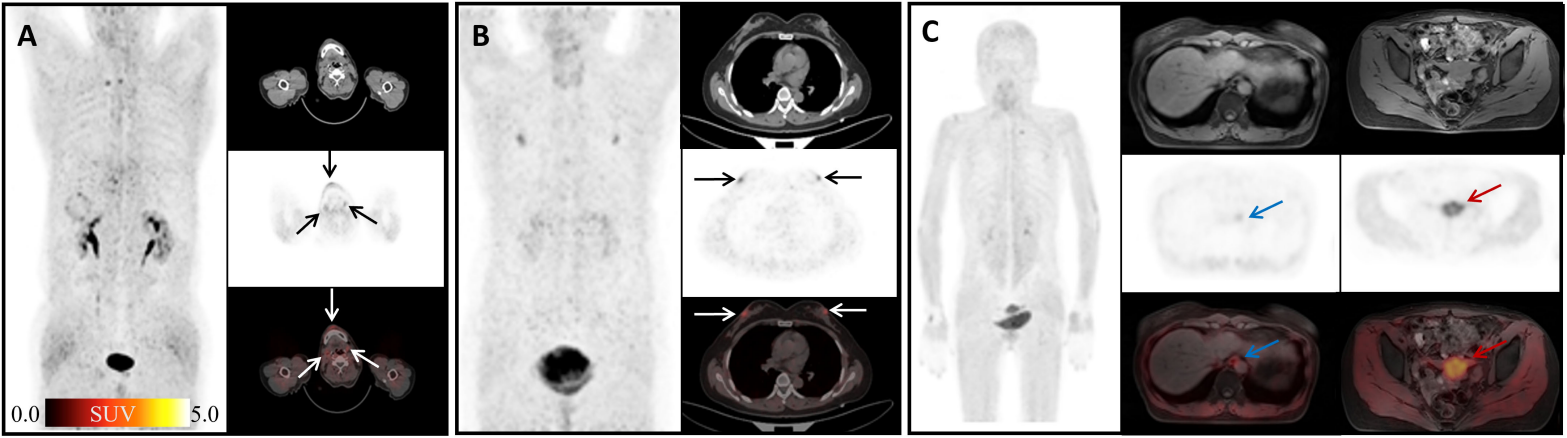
Physiological uptake of ^68^Ga-FAPI-04. (A) A 60-year-old man with hepatic hilar malignancy after the treatment of transcatheter arterial chemoembolization (TACE); the arrows indicate ^68^Ga-FAPI-04 uptake in the gingiva and submandibular gland (SUV_max_ 4.45, 3.79, and 3.64). (B) A 65-year-old woman with microinvasive lung adenocarcinoma 6 months after surgery; arrows show physiological uptake in the nipples with SUV_max_ 4.88. (C) A 55-year-old woman with signet ring cell carcinoma of stomach 2 months after endoscopic submucosal dissection (ESD); blue arrows show physiological uptake of the esophagus (SUV_max_ =3.67), and red arrows show uterus (SUV_max_=13.94).
